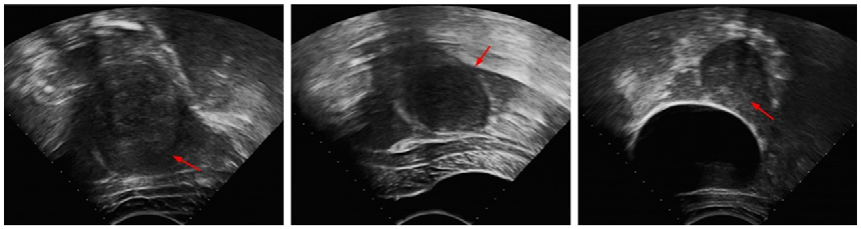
HIFU ultrasound images of uterine fibroids with extremely low SNR, low contrast and weak edges; the red arrows show edges that can easily lead to incorrect segmentation.
